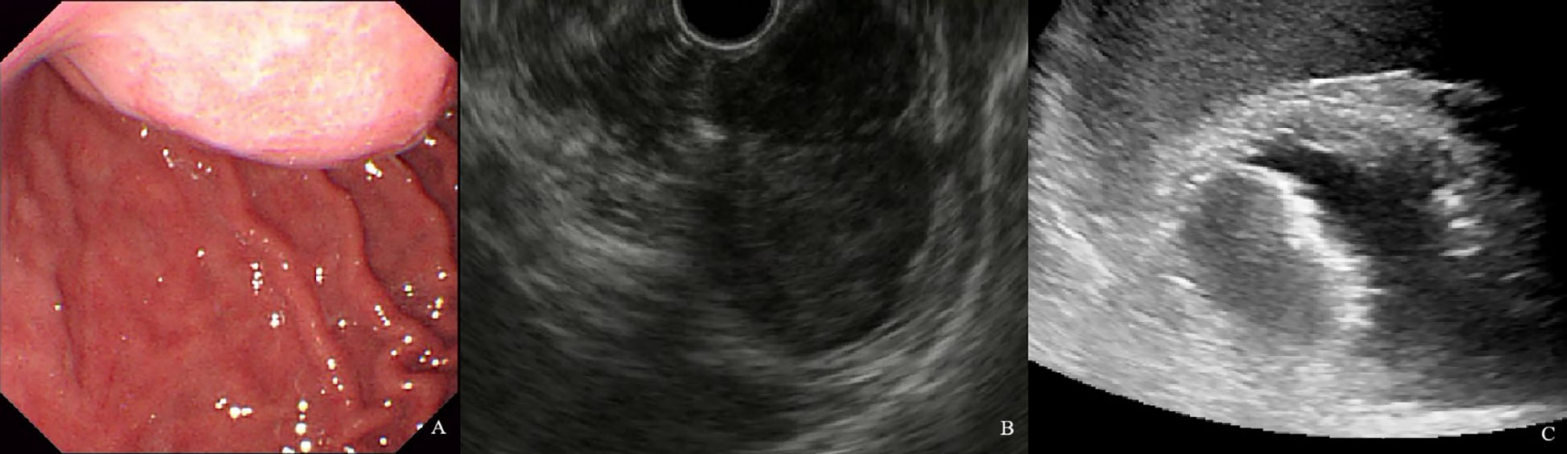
A 54-year-old man with a 4.1×2.6cm GIST of moderate NIH risk stratification. (A) Gastroscopy showed a submucosal elevation with a smooth surface visible in the gastric fundus. (B) Endoscopic ultrasound showed a hypoechoic mass within the fourth layer of the gastric wall. (C) By oral contrast, ultrasound showed a hypoechoic mass in the fourth layer of the gastric body structure with clear borders and homogeneous internal echogenicity.
